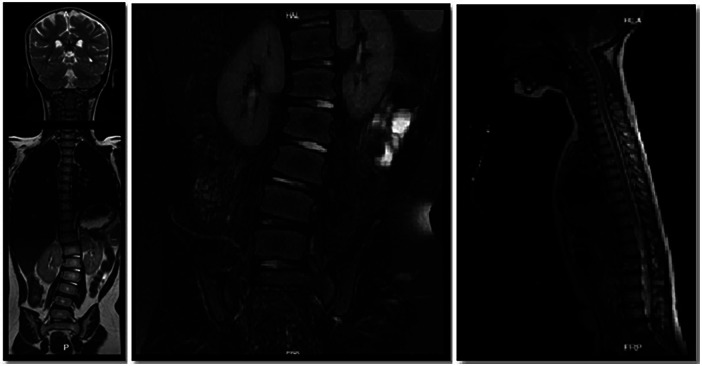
MRI of the spine: the presence of the lumbosacral transition vertebra as the sixth lumbar vertebra is evidenced. There is a right rotoscoliosis with a 22° Cobb angle between L1 and L4. With rectification of the physiological curve of the spine in the dorsal and lumbar region and inversion of the cervical lordotic curve.
